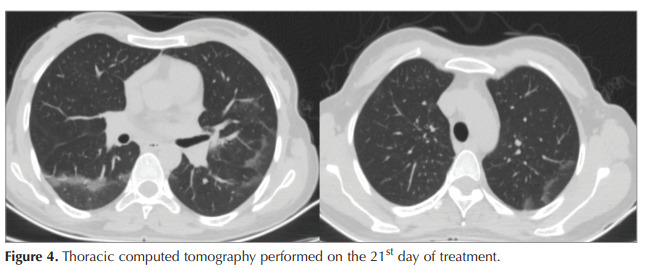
Thoracic computed tomography performed on the 21st day of treatment.
